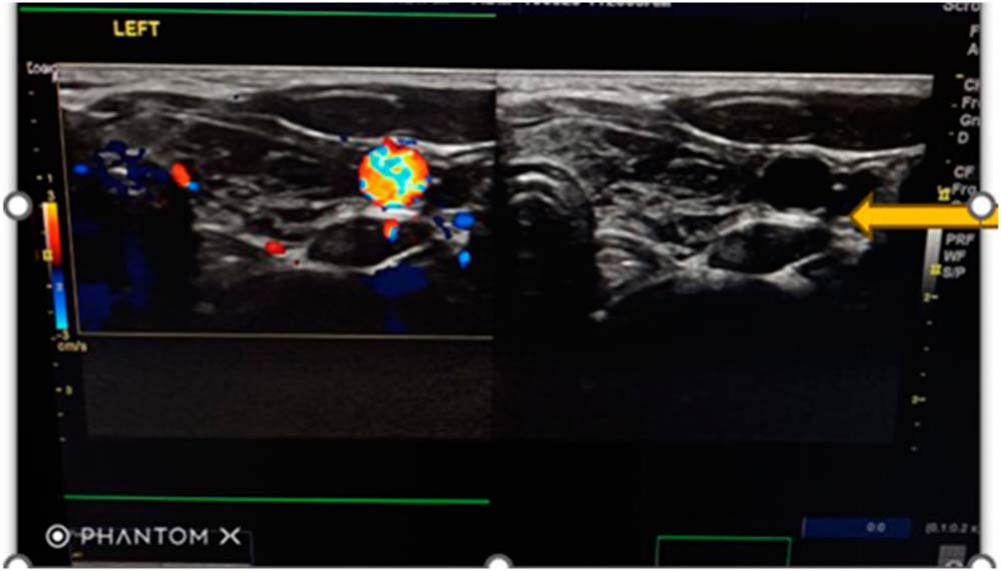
Thyroid ultrasound showing atrophic thyroid gland, normal neck vasculature, yellow arrow.
